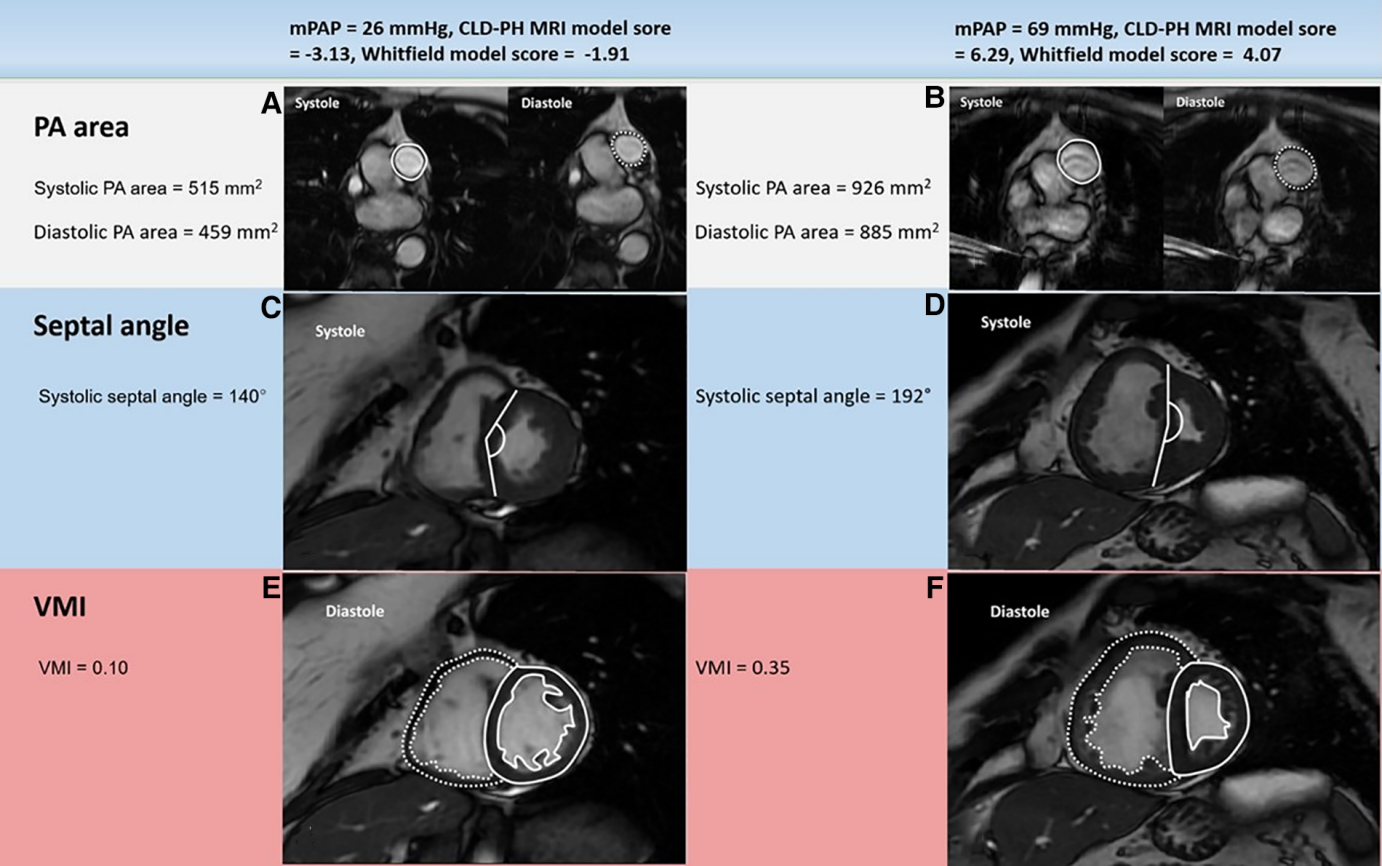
Example cardiac MRI images in patients with mPAP below (left) or above (right) 35 mmHg: PA area (A, B), systolic septal angle (C, D) and VMI (E, F). The patient with severe PH (right) had much larger systolic and diastolic PA areas, systolic septal curvature and VMI than the patient with mild-moderate PH (left). mPAP: mean pulmonary artery pressure; CLD: chronic lung disease; PH: pulmonary hypertension; PA: pulmonary artery; VMI: ventricular mass index; MRI: magnetic resonance imaging.
